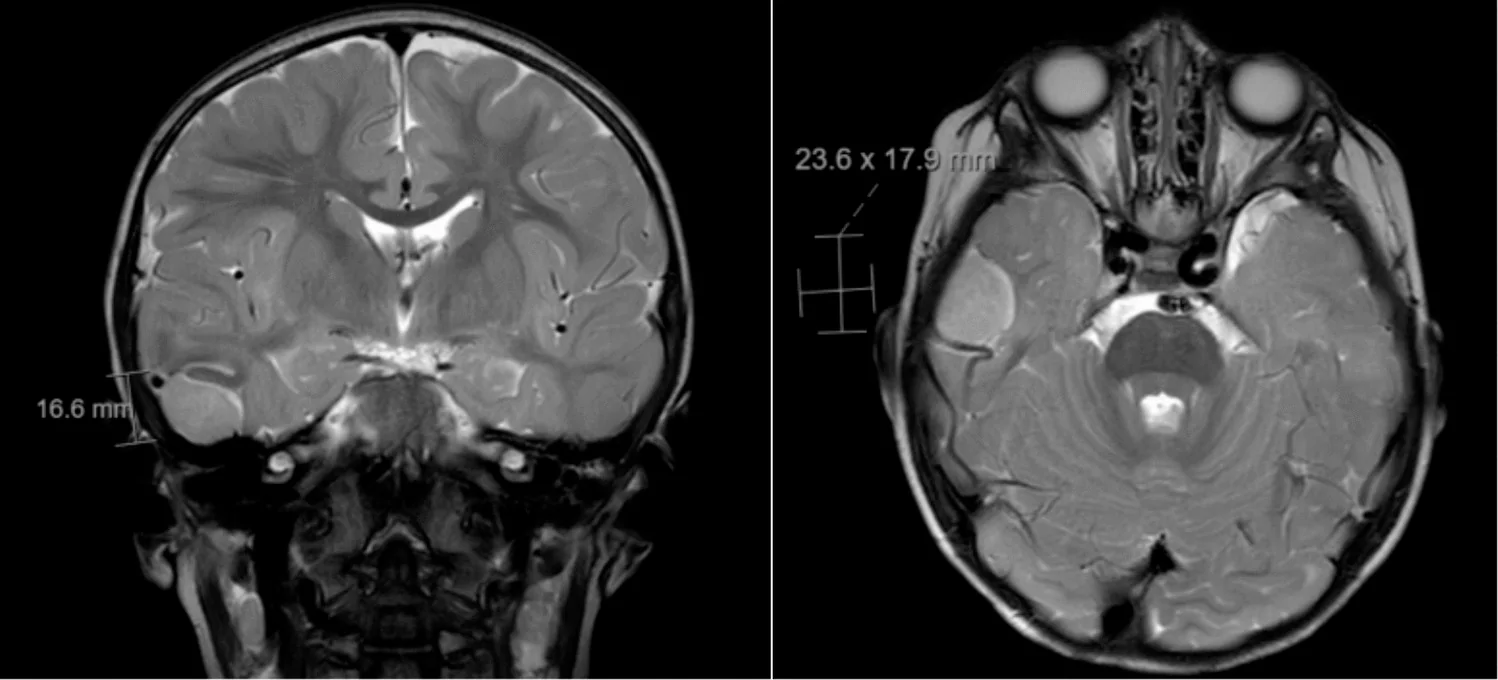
Preoperative MRI of a 16-month-old patient in this cohort demonstrated an extra-axial mass of the right middle cranial fossa with adjacent leptomeningeal enhancement and abnormal signal involving the adjacent right inferior temporal gyrus and sulcus.
